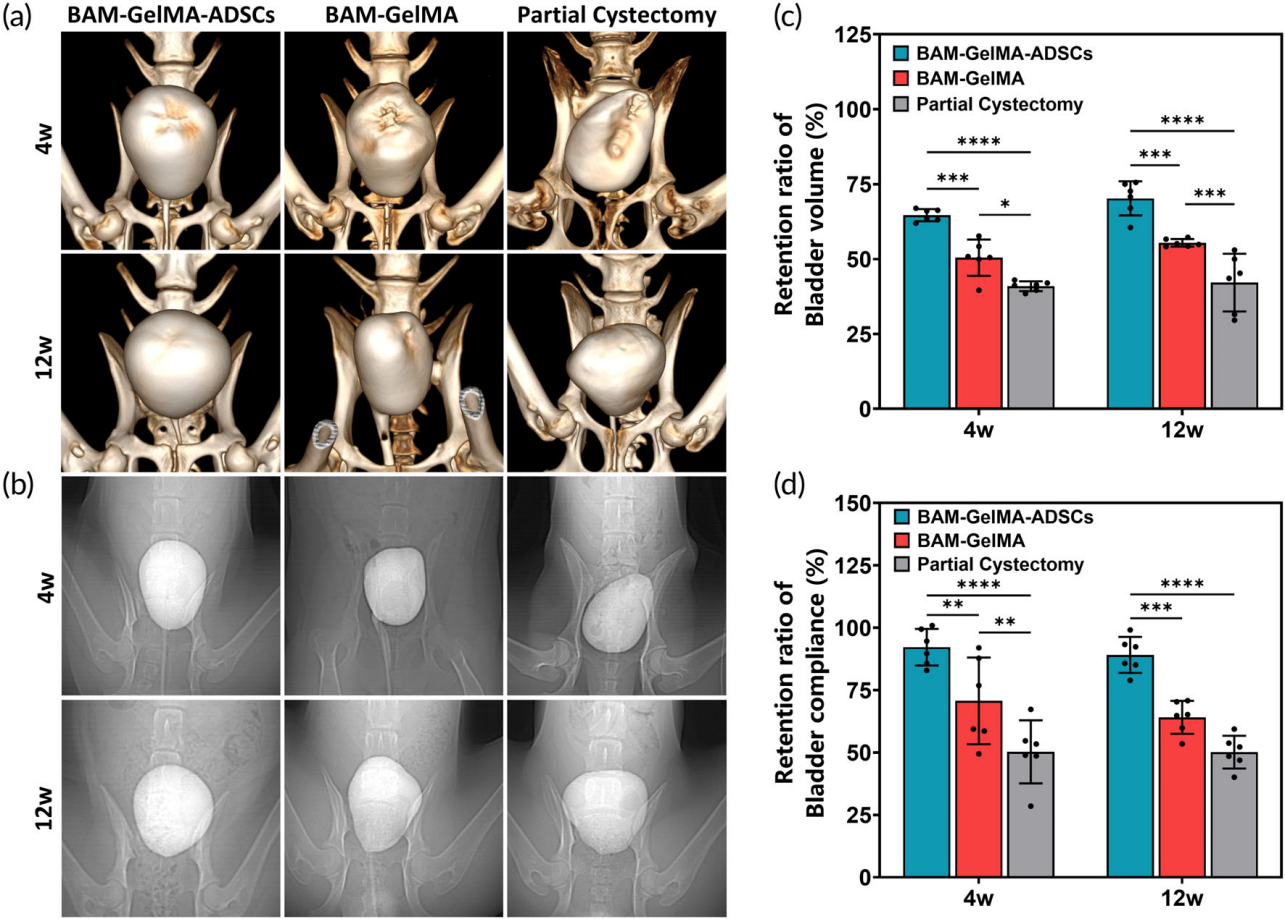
Postoperative imaging and urodynamic examination. (a) Three‐dimensional CT reconstruction of the bladder. (b) Cystography. (c) Urodynamic examination of bladder volume retention ratio (postoperative/preoperative). (d) Bladder compliance retention ratio (postoperative/preoperative). (*n* = 6, 3 per time point) (*p‐*values are calculated using the two‐way ANOVA, p* < 0.05, *p* < 0.01, p* < 0.001, *p* < 0.0001).
